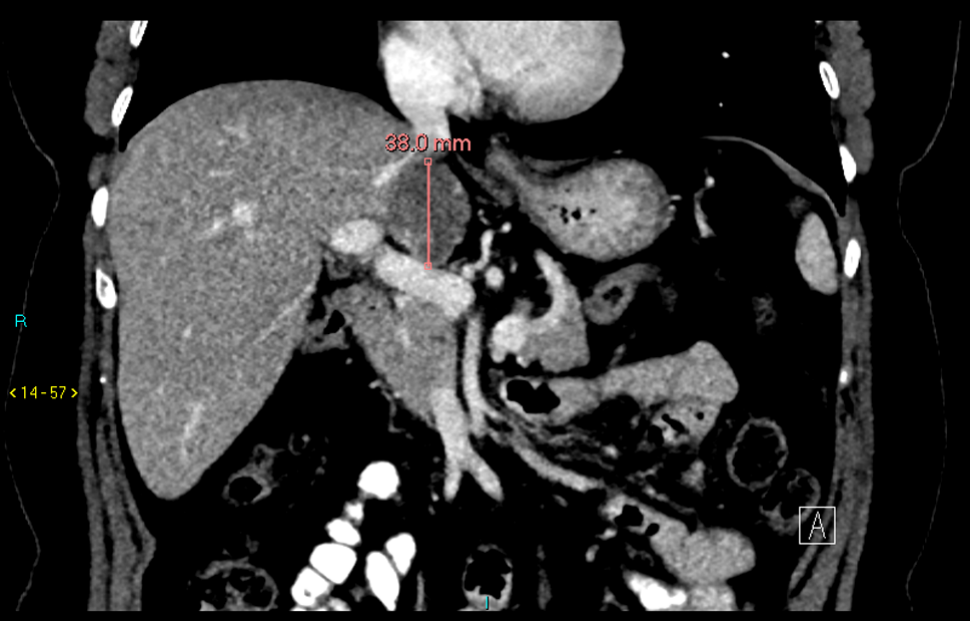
Seven months post-completion SIRT, contrast-enhanced CT showing tumour reduction in size to 38 mm and a volume reduction to 20 cm^3^ and now deemed suitable for surgical resection
